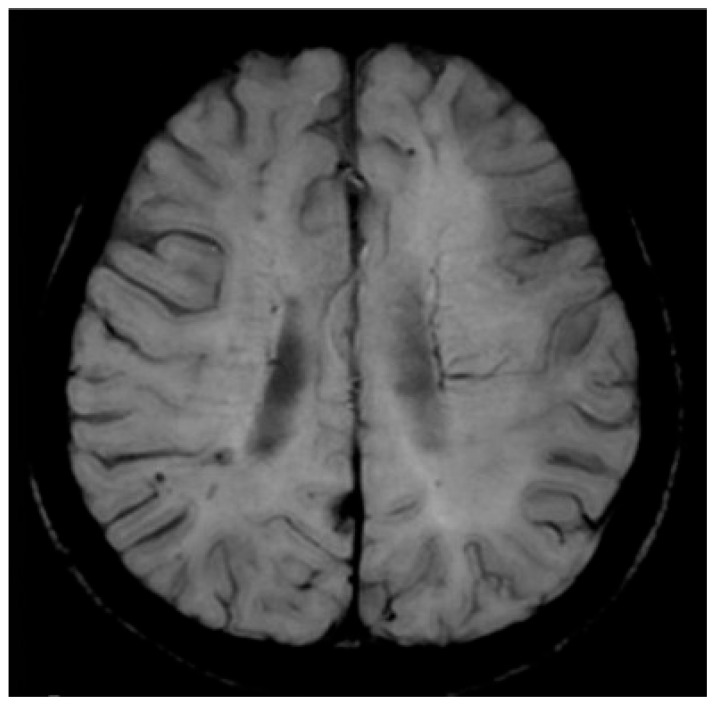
Brain CT imaging showed no acute abnormalities.
